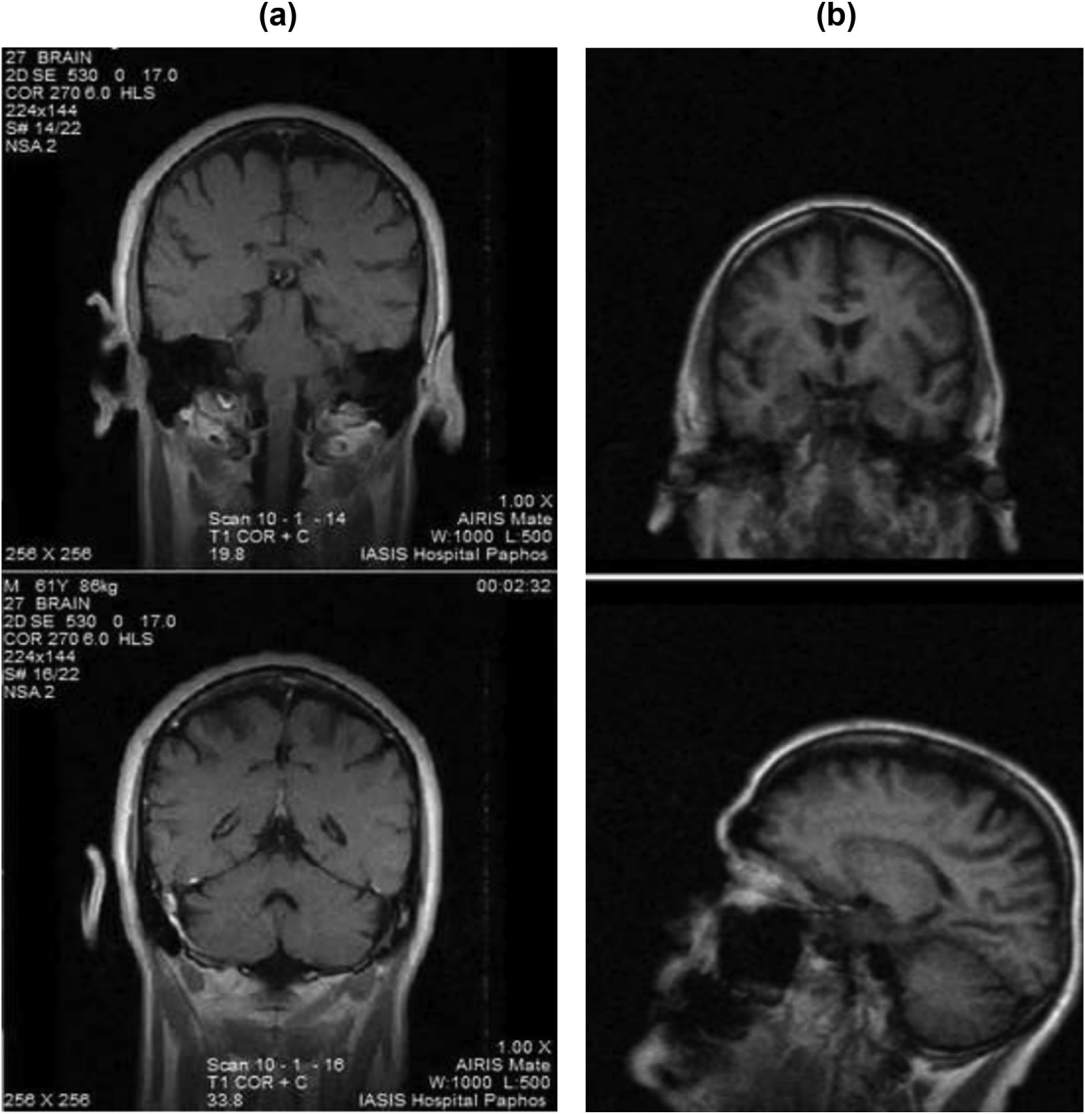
(A) Coronal sections of magnetic resonance imaging (MRI) performed in 2013, showing brain aging and partially empty sella turcica, (b) Coronal and sagittal sections of MRI performed in 2020, showing mild cerebral atrophy/aging more pronounced in the curvatures of the cerebral hemispheres.
